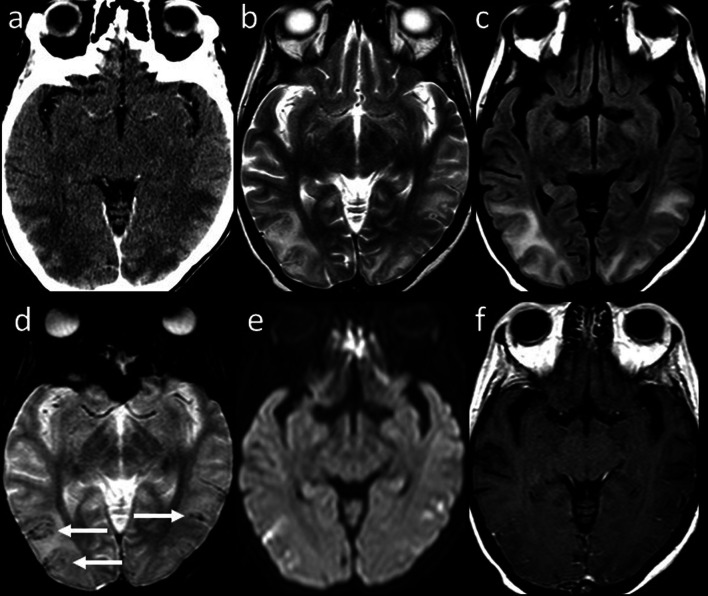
Posterior Reversible Encephalopathy Syndrome. Axial contrast-enhanced CT image (a) of a 40-year-old woman with metastatic cervical carcinoma treated with multiple ChT agents including Cisplatin and 5-Fluorouracil shows bilateral parieto-temporo-occipital, subcortical and cortical, hypodense and non-enhancing foci. Axial T2-weighted (b) and FLAIR (c) images reveal corresponding hyperintense areas. Axial GRE T2*-weighted (d) image shows cortical and focal hypointense foci (arrows) related to microhemorrhages with neither diffusion abnormality on DWI (e) nor pathologic contrast enhancement on axial post-contrast T1-weighted image (f)
